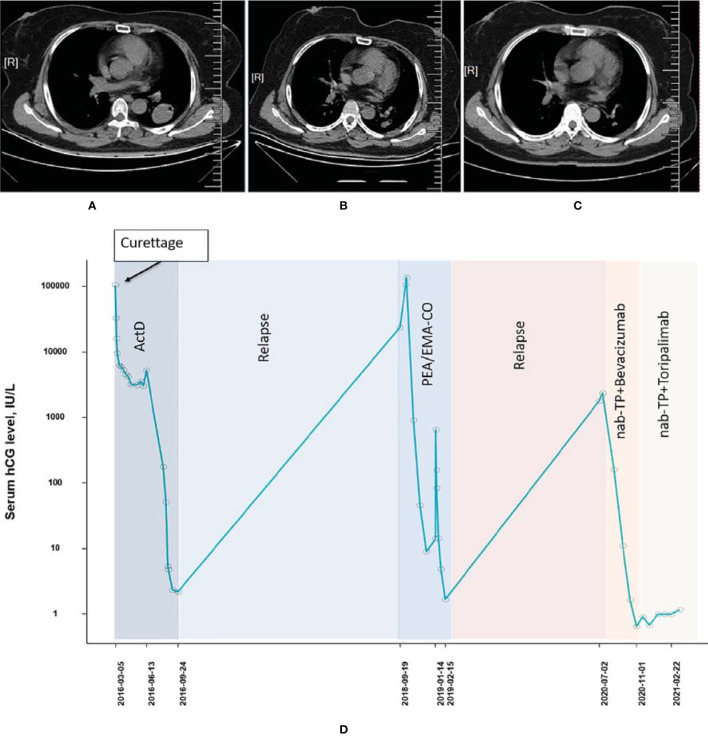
Computed tomography imaging (A) before pulmonary metastasis, (B) before combined treatment of PD-1 and chemotherapies, and (C) after combined treatment of PD-1 and chemotherapies (patient 3). (D) The concentration of the β-hcg level since the diagnosis of choriocarcinoma (patient 3).
